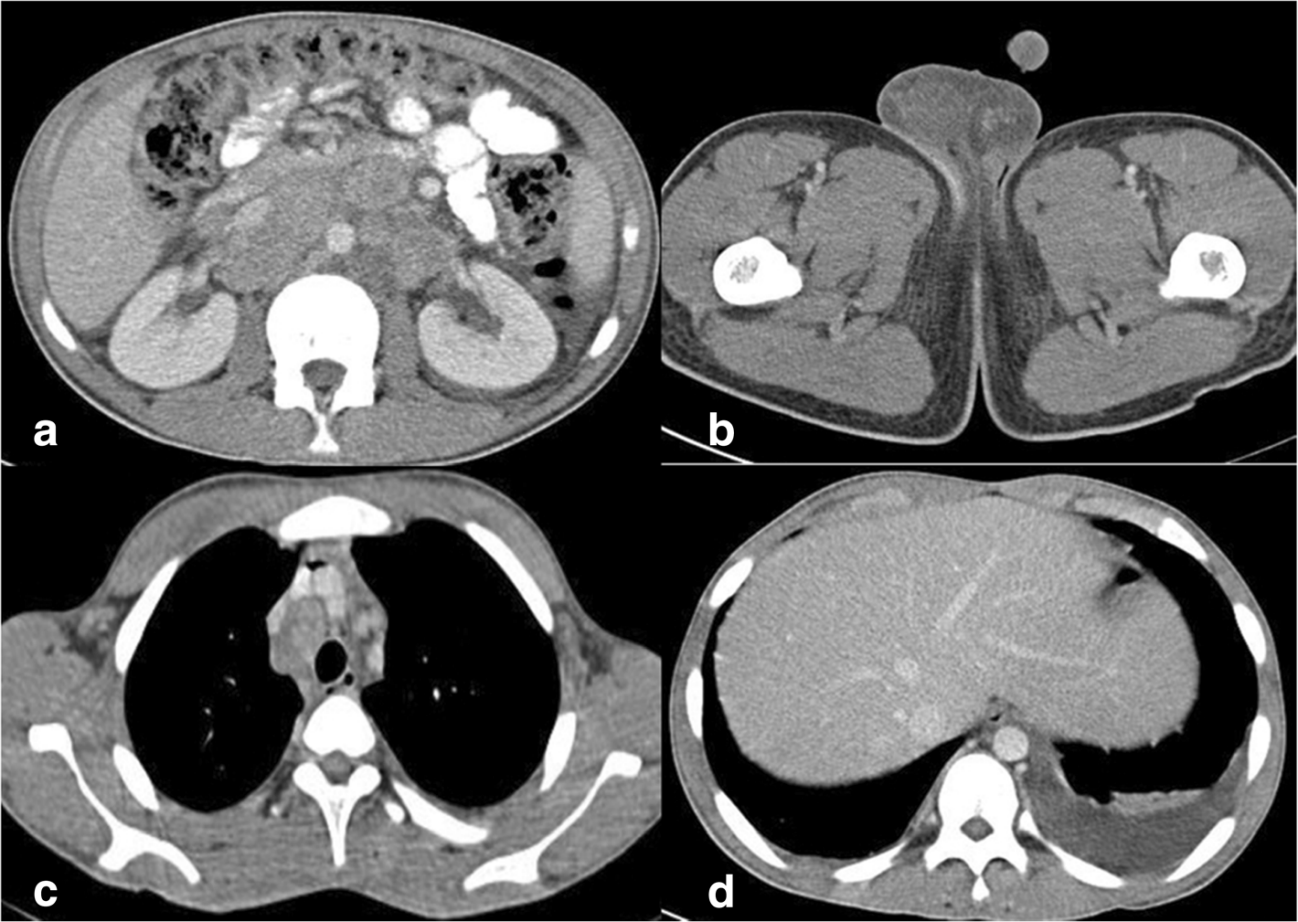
Contrast-enhanced computed tomography of the abdomen, pelvis, and chest. a Retroperitoneal lymphadenopathy. b Right testicular enlargement. c Mediastinal lymphadenopathy. d Left pleural effusion
